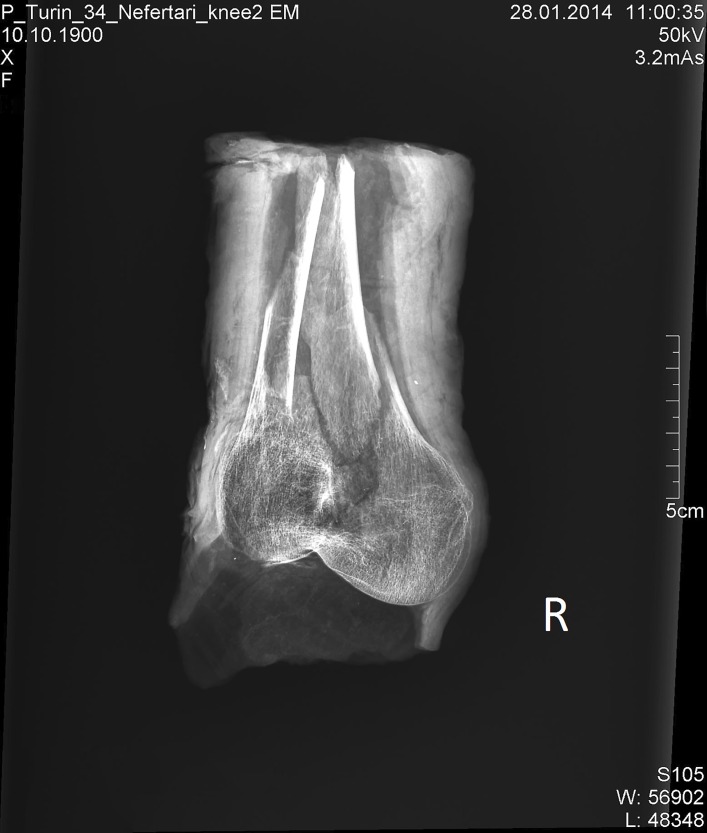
X-ray of right distal femur, pa.
